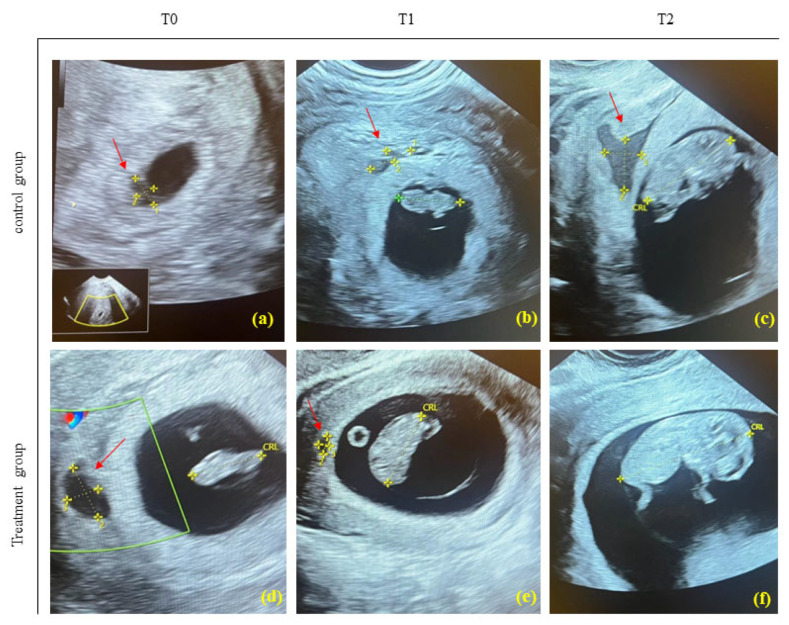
Representative ultrasounds of SCH (red arrow) of patients following or treatment with P4 (control group) (a–c) and HMWHA in association with ALA, magnesium, vitamin B6, and vitamin D (treatment group) (d–f). Ultrasound at T0, baseline; at T1 (1 week of treatment); at T2 (2 weeks of treatment). In the treatment group with HMWHA in association with ALA, magnesium, vitamin B6, and vitamin D, subchorionic hematoma (f) was no longer detectable at T2, unlike the control group (c). CRL = Crown-Rump Length.
